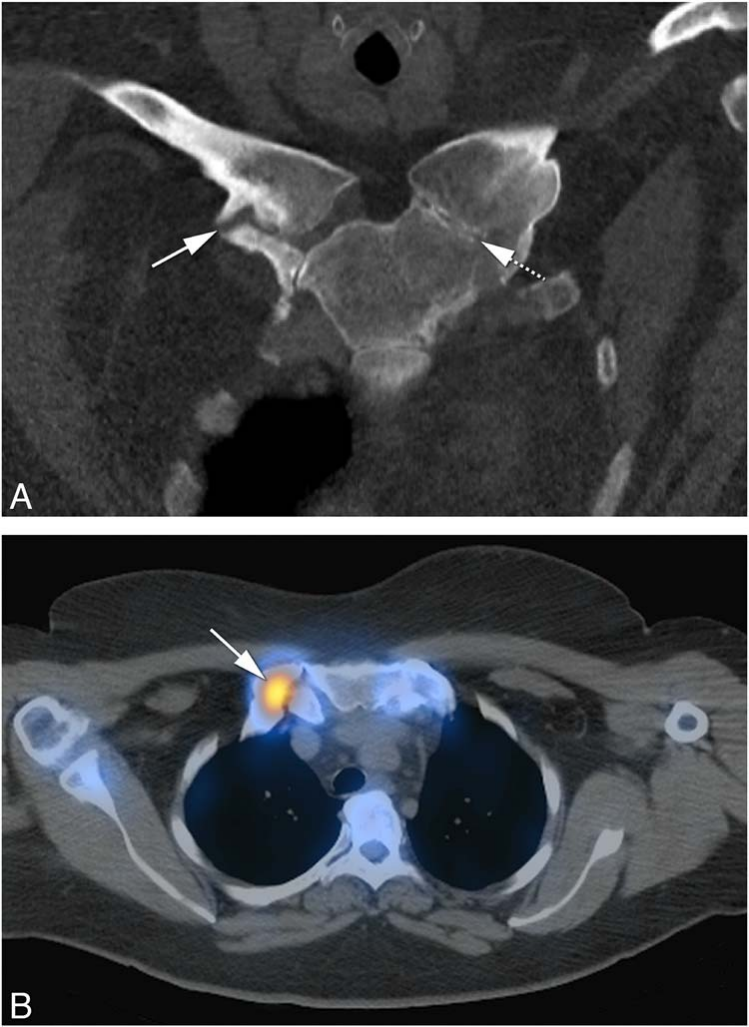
(A) Calcification of the right costoclavicular ligament (arrow) and complete ankylosis of the left costoclavicular joint (dashed arrow). (B) Focal increased uptake at the medial end of the right clavicle and first rib indicating focal active bone formation (arrow). Single photon emission computed tomography of the SCC region showing normal uptake at the left clavicle and sternal manubrium reflecting a more chronic course of the disease at that site.
